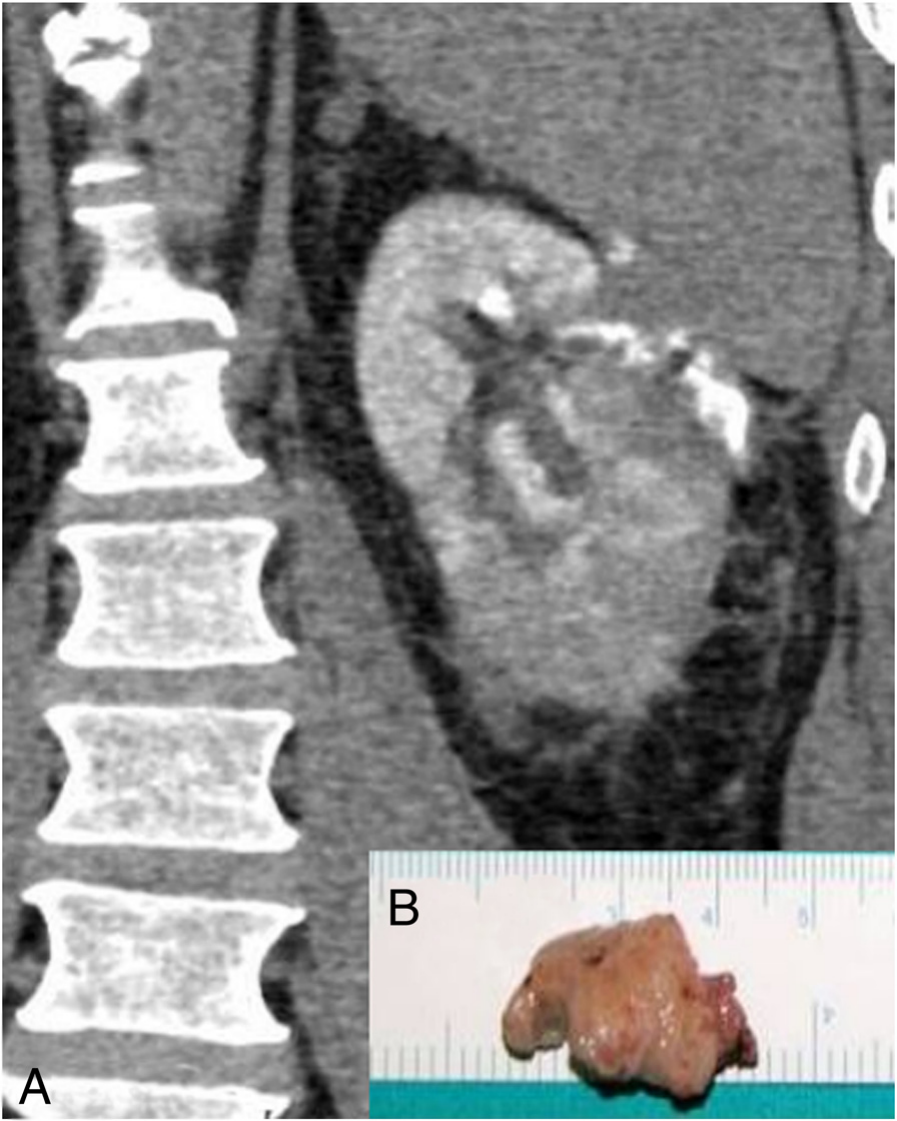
Treatment of case n. 5. A) Uro-CT scan following repeated embolization of a fistula of the upper calyx caused by thermal damage during laparoscopic enucleoresection of RCC. The radiopaque urine flows around the calyceal plug and reaches the external kidney surface. B) Inset: cyanoacrylate cast of the upper calyx removed at nephrectomy.
